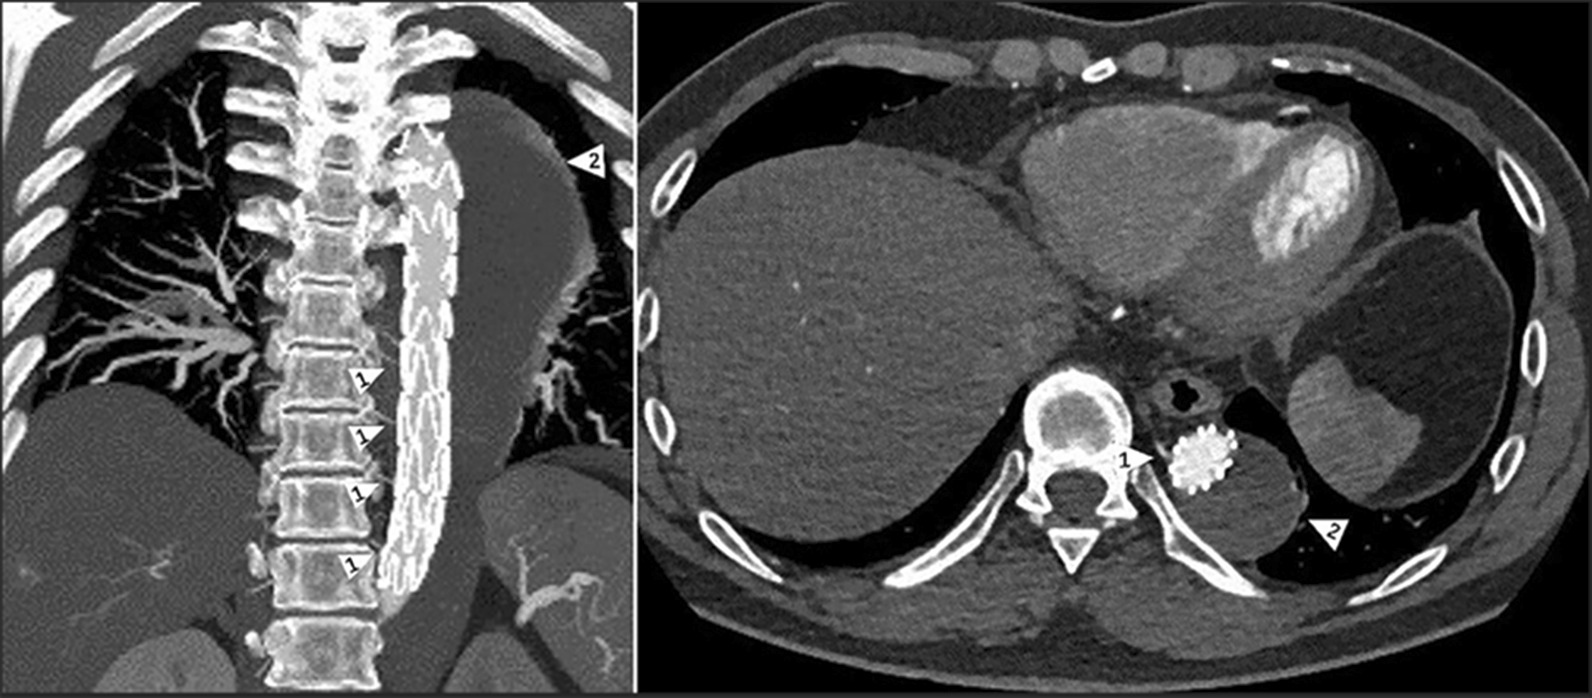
CTA review postoperative 1 week: Talos stent completely isolated the false lumen, and the blood supply of the intercostal artery was patched. Arrow 1 indicates the intercostal artery and arrow 2 indicates the false lumen
